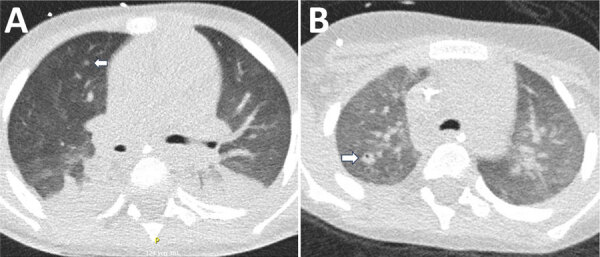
Chest computed tomography scan of a 4-year-old male patient with acute myeloid leukemia in study of *Bjerkandera* spp. pulmonary infection in immunocompromised hosts, Germany. The patient experienced fever unresponsive to antimicrobial treatment. A) Imaging revealed nodular infiltrates and surrounding ground-glass opacities in both lungs (arrow). *Bjerkandera* spp. was identified from tracheal aspiration. B) Follow-up computed tomography scan after 4 weeks demonstrated regressive nodular lesions and the formation of a cavity in the right upper lobe (arrow).
